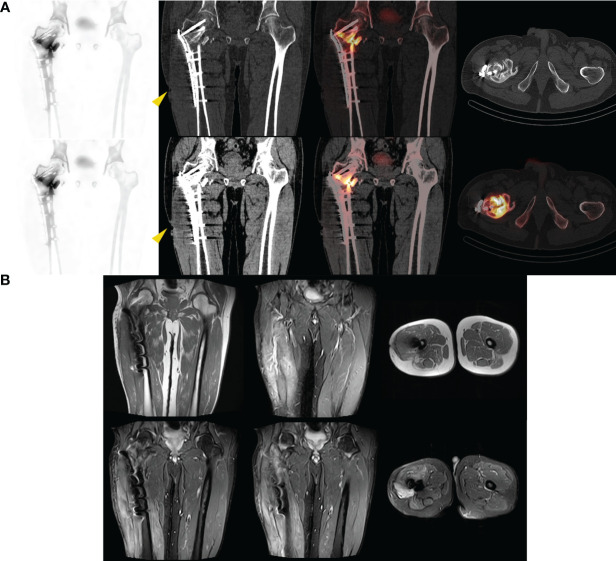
Male. 61y. After 9 months of open reduction and internal fixation for intertrochanteric fracture of the femur. Pain at the right thigh wound, with purulent discharge visible. A sinus formation can be seen in the middle section of the scar on the left thigh after surgery, with a small amount of secretion oozing out. WBC: 4.79×10^9^/L; LYM%: 32.6%; MONO%: 6.7%; NE%: 54.1%; hs-CRP: 2.11mg/L. Bacterial culture suggests Escherichia coli. (A) SPECT/CT suggests that after the right proximal femur surgery, there are bone changes in the surgical area accompanied by abnormal bone metabolism, as well as low-density soft tissue shadows and sinus formation (yellow arrow), suggesting an infectious lesion. (B) MRI considers changes after internal fixation surgery, resulting in swelling of the thigh soft tissue. Edema of subcutaneous soft tissue on the outer side of the right thigh.
